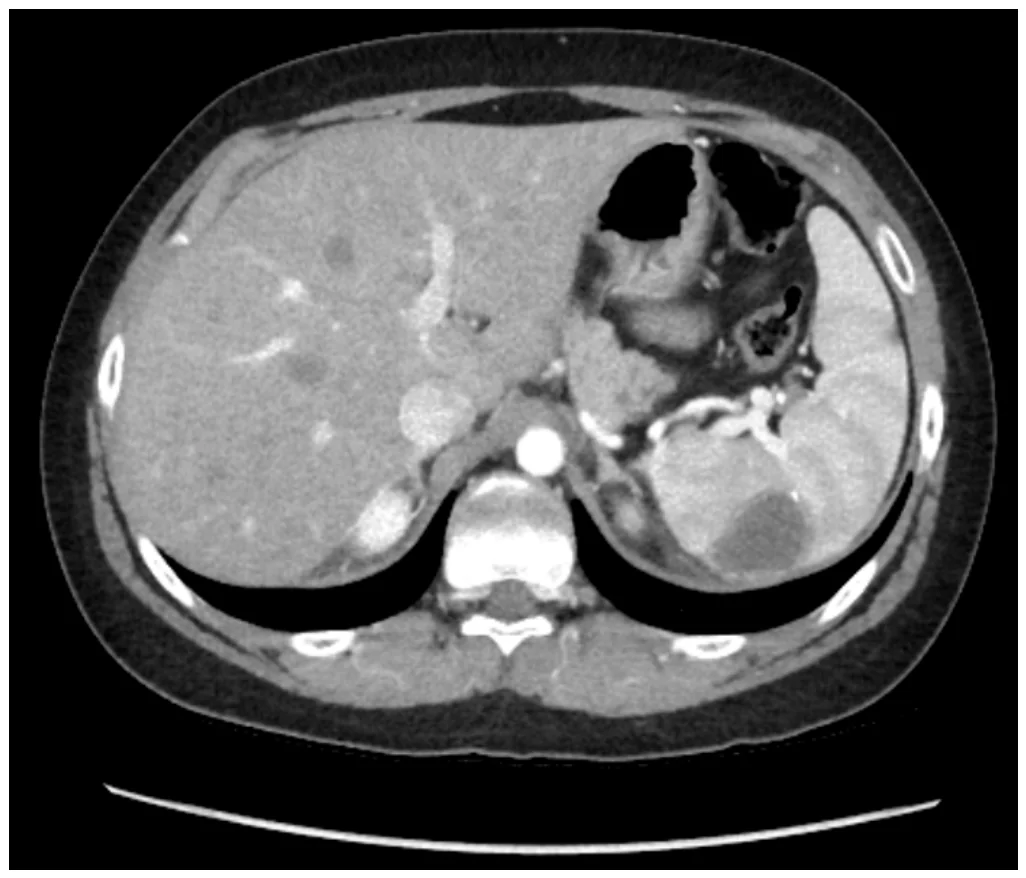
Contrast-enhanced computed tomography of the abdomen showing, at the upper pole of the spleen, an ovalar cystic lesion of fluid density (~3.7 × 2.5 cm) with thin peripheral calcifications and a regular enhancing pericystic rim, compatible with a splenic hydatid cyst.
